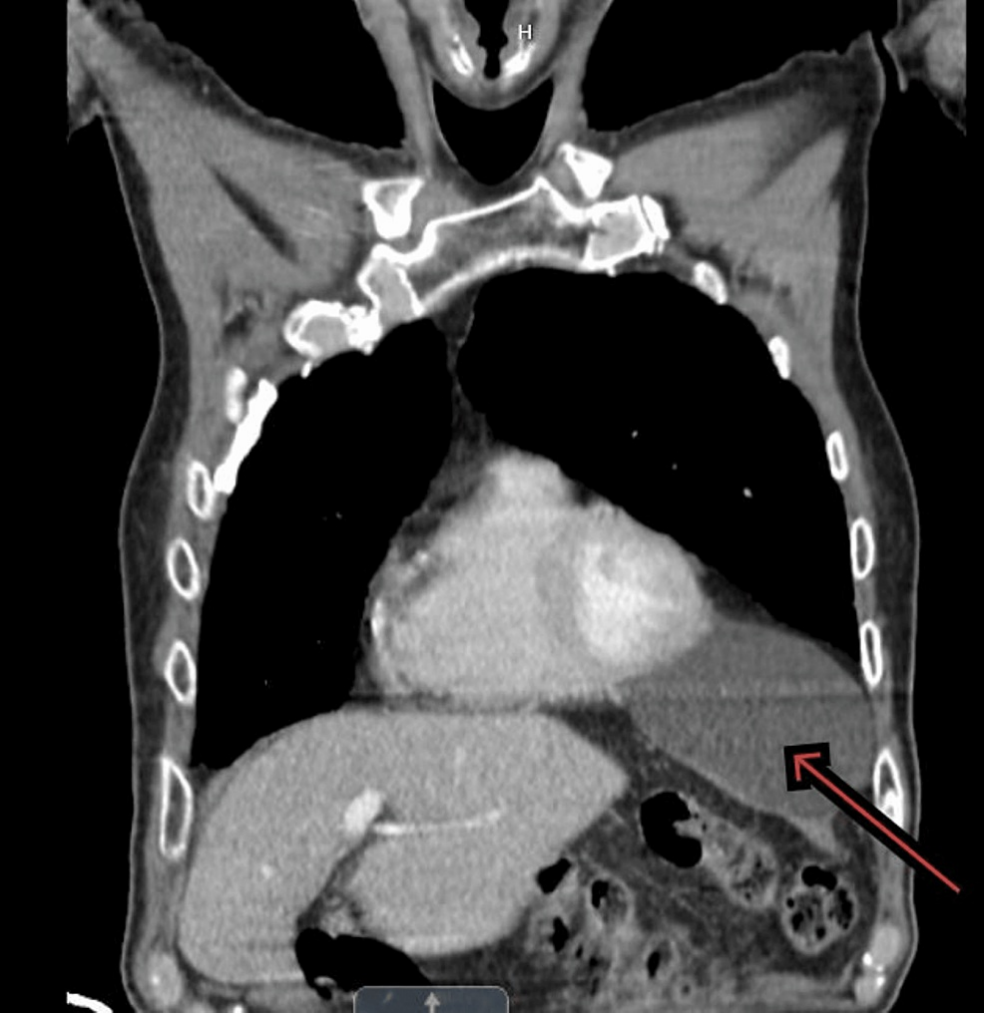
CT scan of chest in coronal section showing a big pericardial cyst (red arrow)
